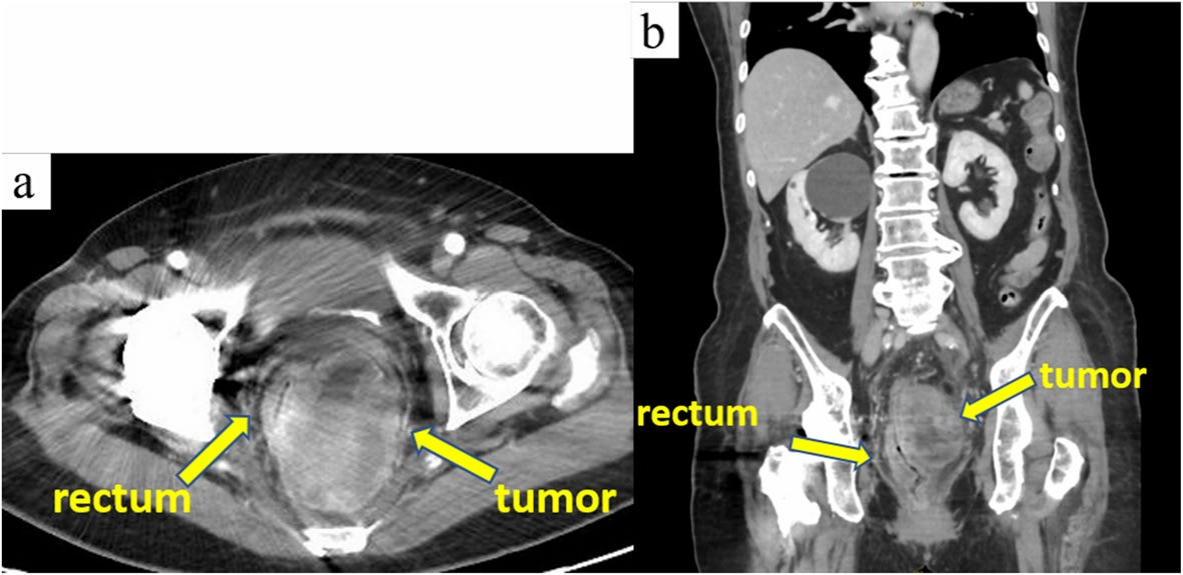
Contrast-enhanced computed tomography revealed a 53 × 58 × 75 mm mass with well-defined boundaries and a heterogeneous contrast effect on the left side of the rectum. Fluid accumulation was observed in the center of the mass, which suggested necrotic tissue
